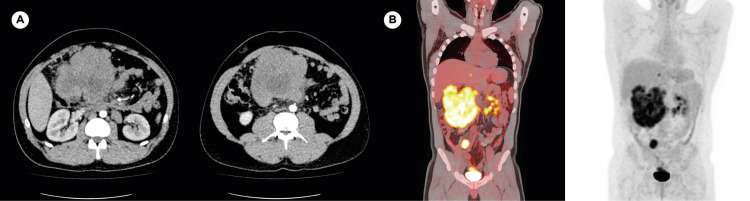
Imaging studies A) Left: contrast CT axial planes show large, heterogeneous mass occupying the epi- and mesogastrium, as well as periaortic and interaortocaval lymphadenopathies. B) Right: PET and fusion (PET-CT) images: coronal plane maximum intensity projection showed a large, lobulated mass in mesogastrium with SUVmax of 9.7, hypodense center due to central necrosis, pelvic implant (posterosuperior to the bladder), and right common iliac metastatic adenopathy CT: computed tomography; PET: positron emission tomography
